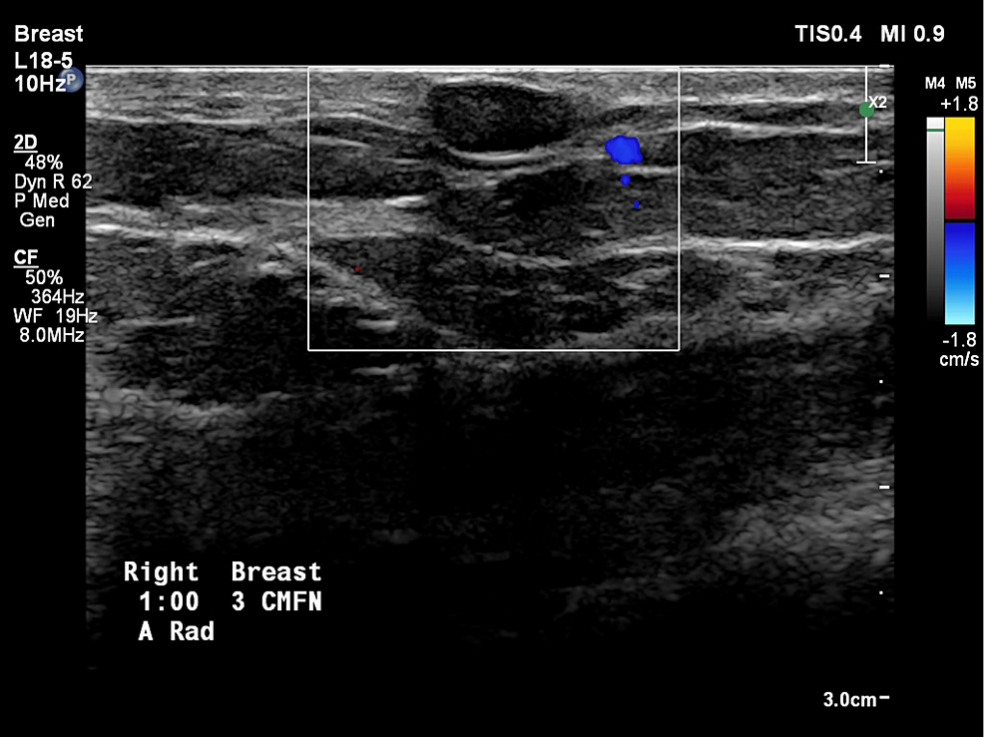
Doppler ultrasound image of oil cysts in the patient’s right breast depicting a circumscribed cyst in the 1 o’clock position, 3 cm from the nipple.
